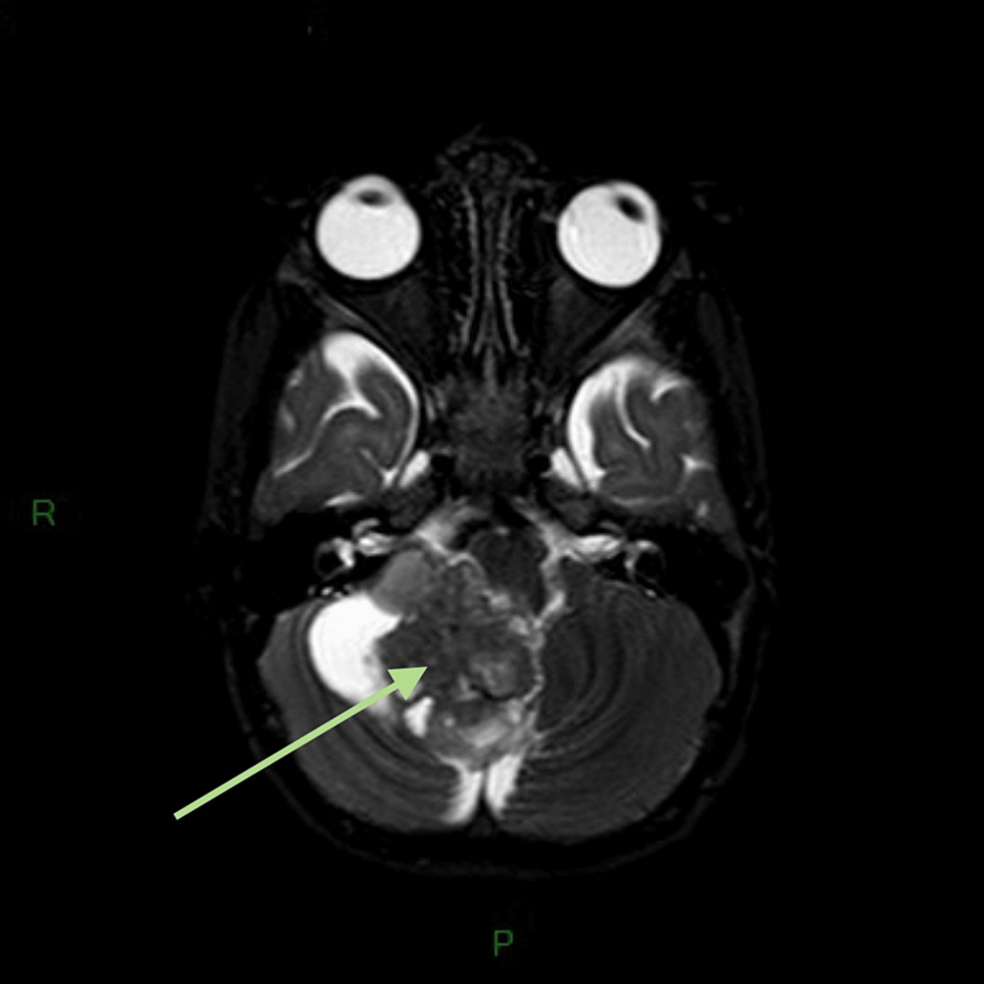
Preoperative axial T2-weighted STIR MRI. The image shows a round heterogeneous mass in the posterior fossa compressing the fourth ventricle with consequent obstructive hydrocephalus. R, right side; P, posterior; STIR, short-tau inversion recovery
